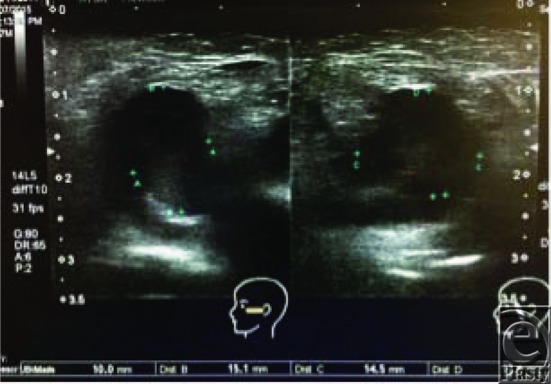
Ultrasound showing heterogeneous mass on corresponding left cheek.
